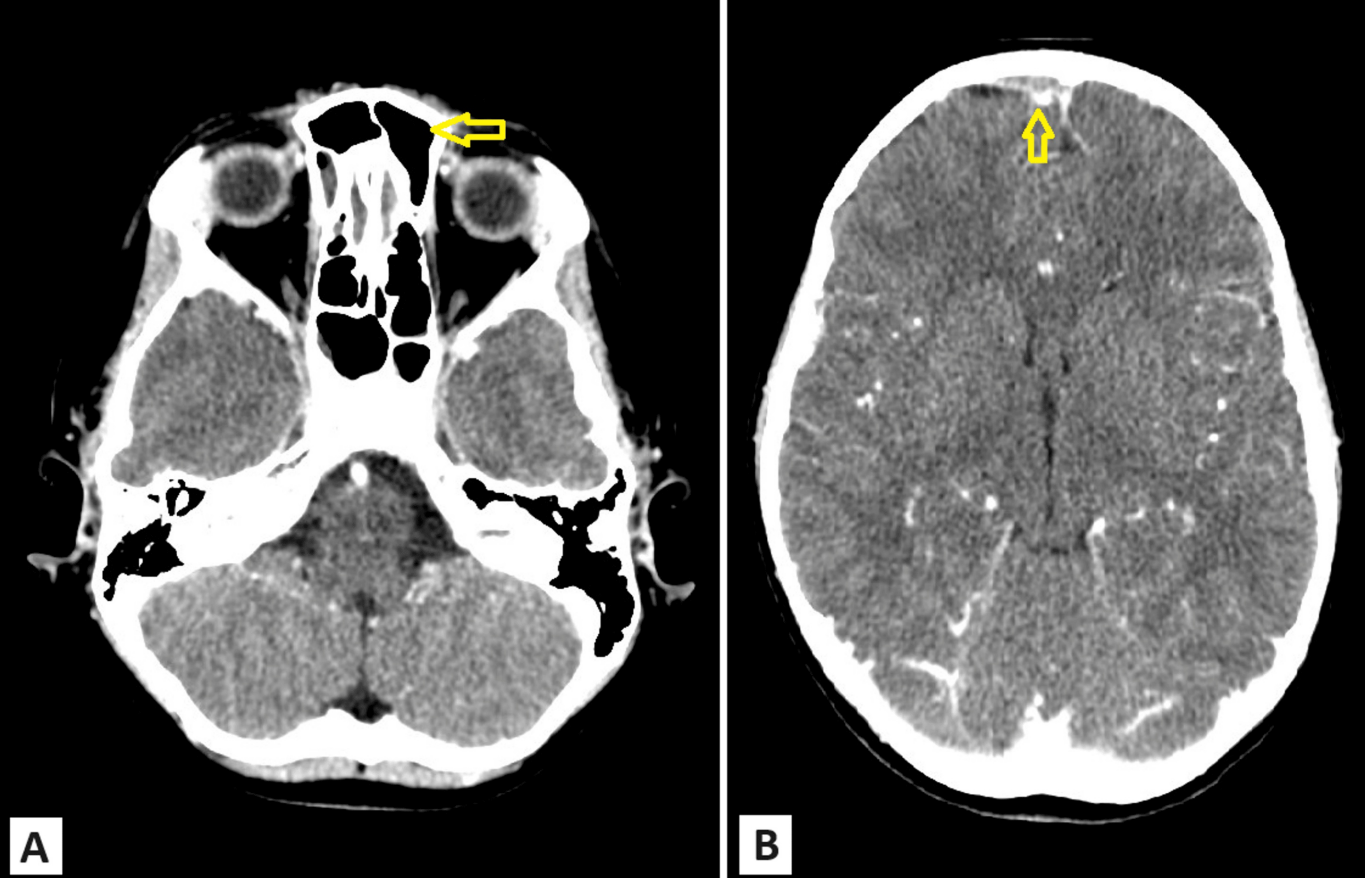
Follow-up brain CT scan with contrast done after two weeks of treatment. Panel (A) shows resolving frontal sinusitis. Panel (B) shows an extra-axial soft tissue lesion in the anterior frontal region with peripheral enhancement, which has significantly reduced in size compared to the previous study. Horizontal arrow: resolving left frontal sinusitis; vertical arrow: reduction in size of extra-axial collection
